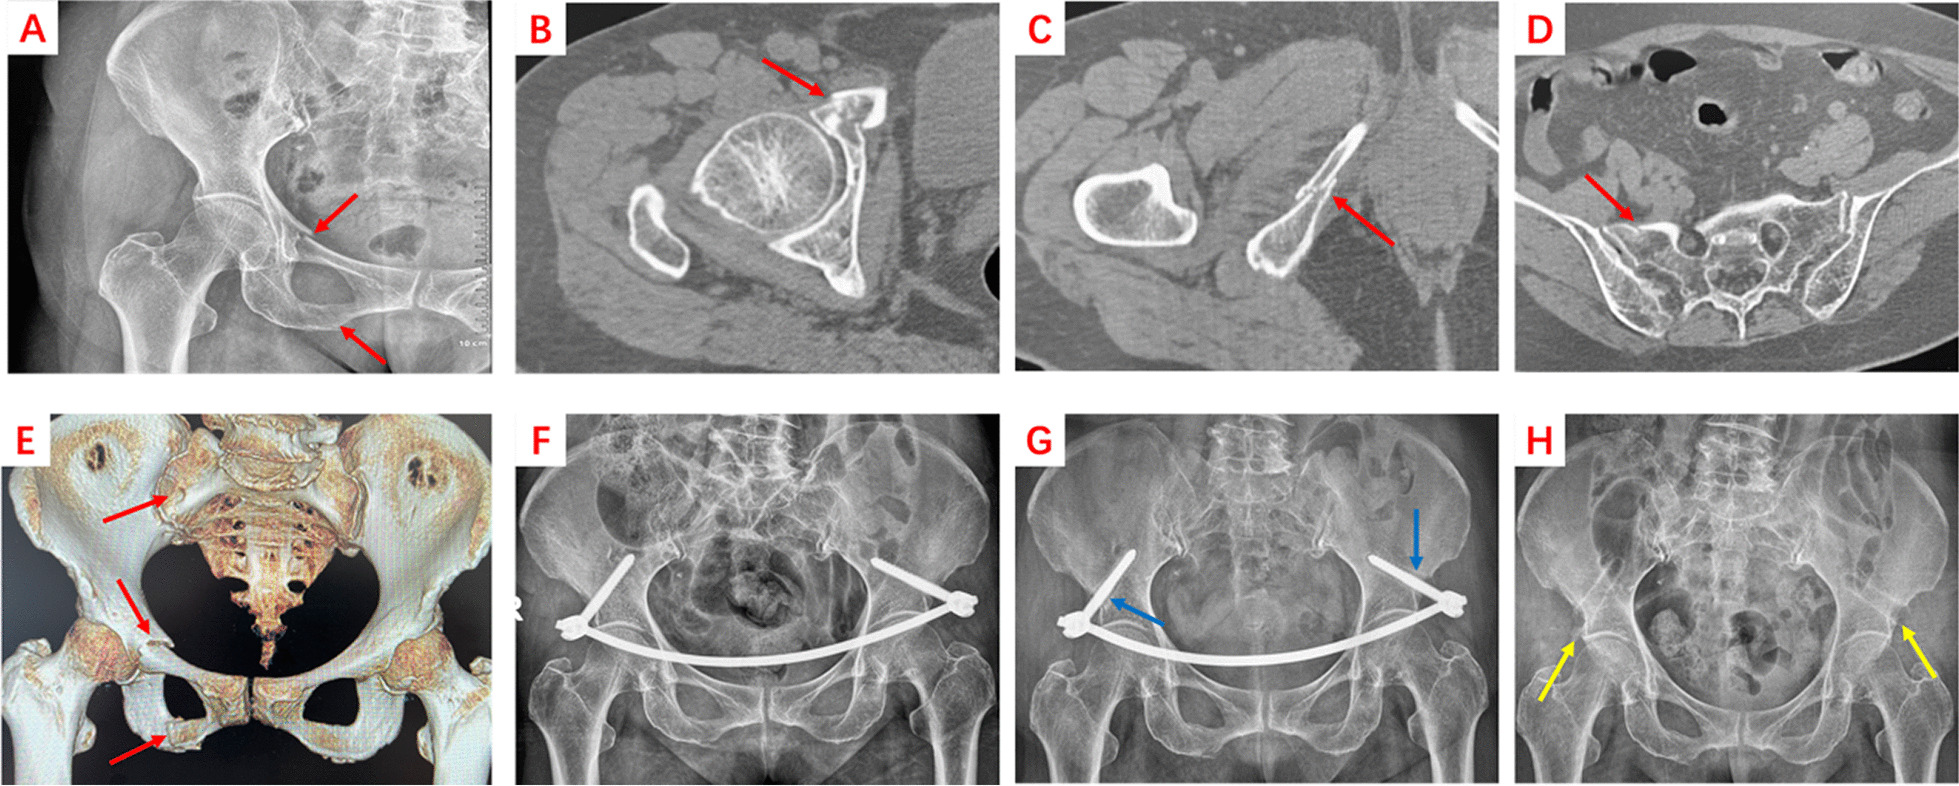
An 86-year-old patient with LC1 pelvic fracture underwent surgery with INFIX. A X-ray examination on admission showed fractures of the upper and lower rami of the right pubic bone (red arrows). B–E CT scan and three-dimensional reconstruction indicated incomplete fractures of the right superior and inferior ramus of the pubis and ipsilateral sacrum (red arrow). F X-ray examination taken after INFIX fixation. G At 4 months after surgery, the X-ray image showed that the fracture had healed and there was an empty shadow around the screw, indicating that the screw was loose (blue arrow). And INFIX fixation was subsequently removed. H At 13 months after surgery, the X-ray image showed a small amount of heterotopic ossification was seen in the anterior inferior iliac spine (yellow arrow)
